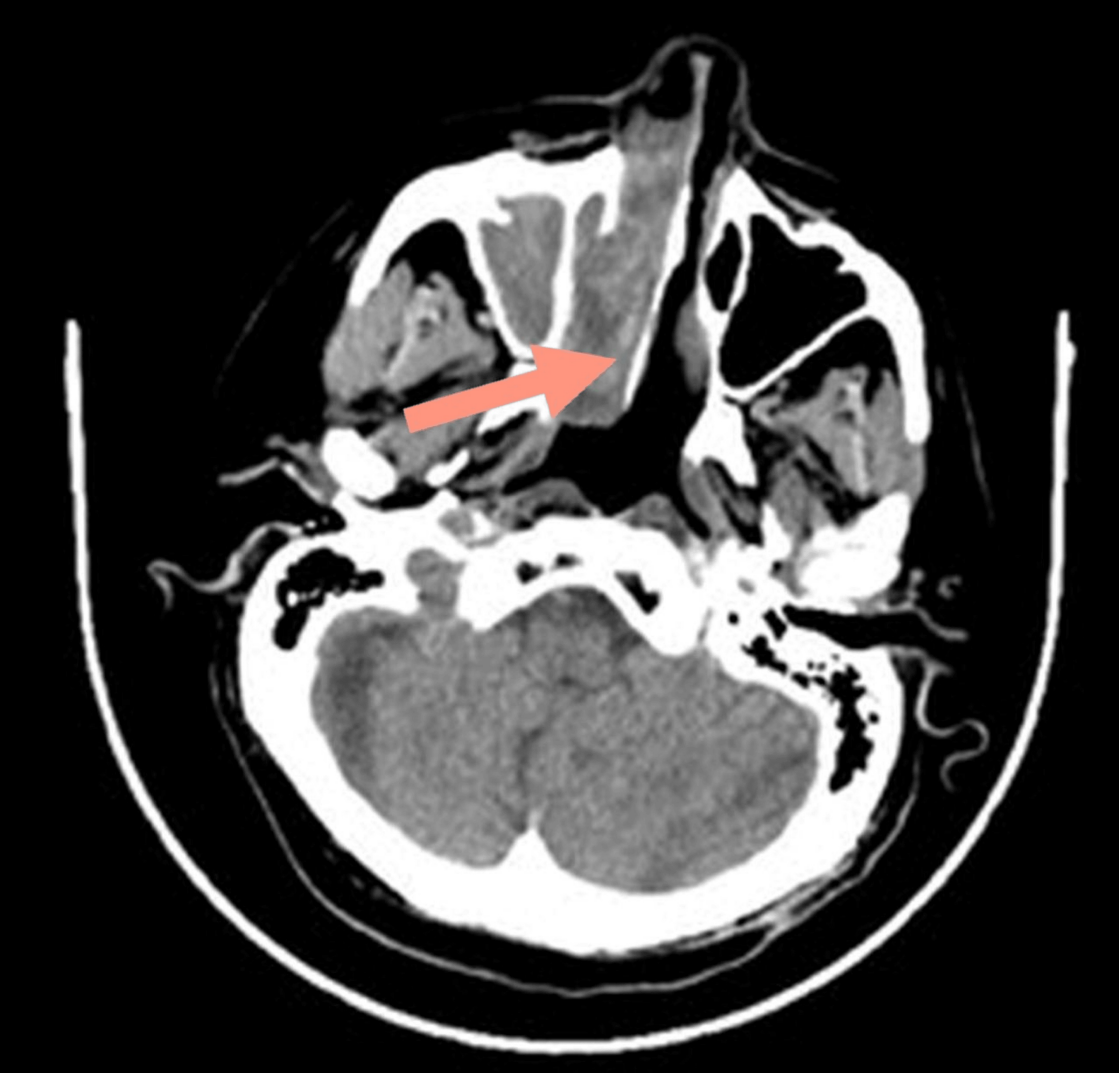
Axial CT with contrast showing right nasal cavity mass extending into the nasopharynx (arrow).
